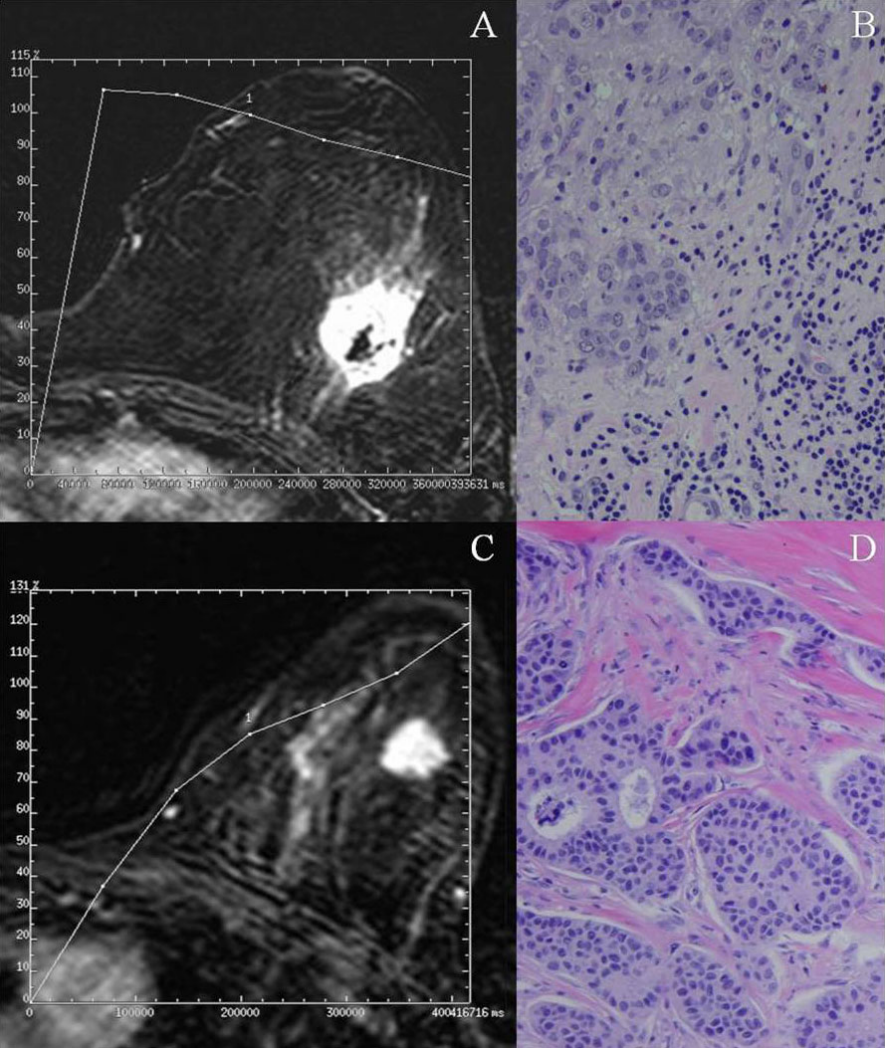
Breast MR image acquired in a 45-years-old patient with a palpable mass in the left upper outer quadrant. The axial postcontrast subtracted image (9.9/4.2; flip angle, 10°) depicts a lesion with irregular margin of mass and heterogeneous enhancement (arrow). The time-signal intensity curve of this shows a type III (washout) curve. (A). Microphotography of the same tumor showing a peritumoral inflammation (arrows). 200×. (B). Breast MR image acquired in a 63-years-old patient with a palpable mass in the left upper outer quadrant. The axial postcontrast subtracted image (9.9/4.2; flip angle, 10°) depicts a lesion of irregular margin of mass (arrow). The time-signal intensity curve of this shows a type I curve. (C). Microphotography of the same tumor showing no a peritumoral inflammation. 100×. (D).
